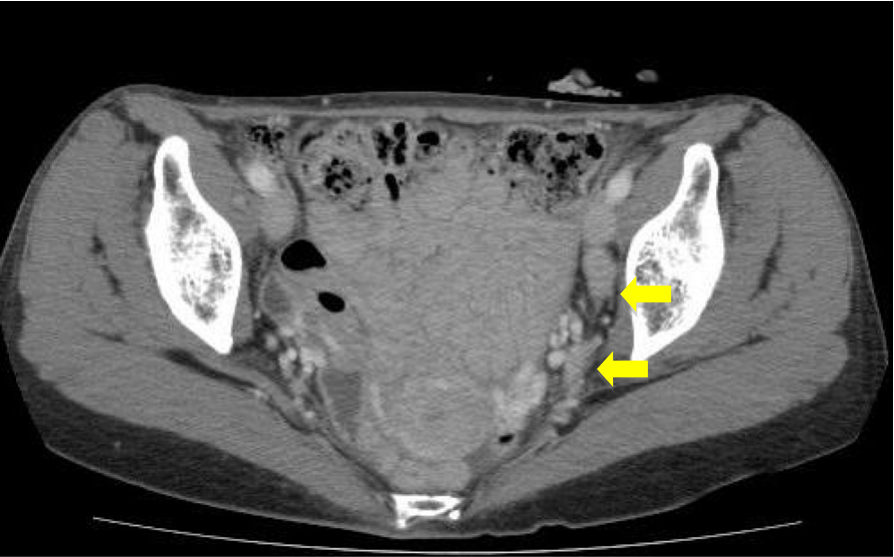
Abdominal CT scan 18 months after surgery showed an enlarged lymph node at the left external and internal iliac regions (arrow)
